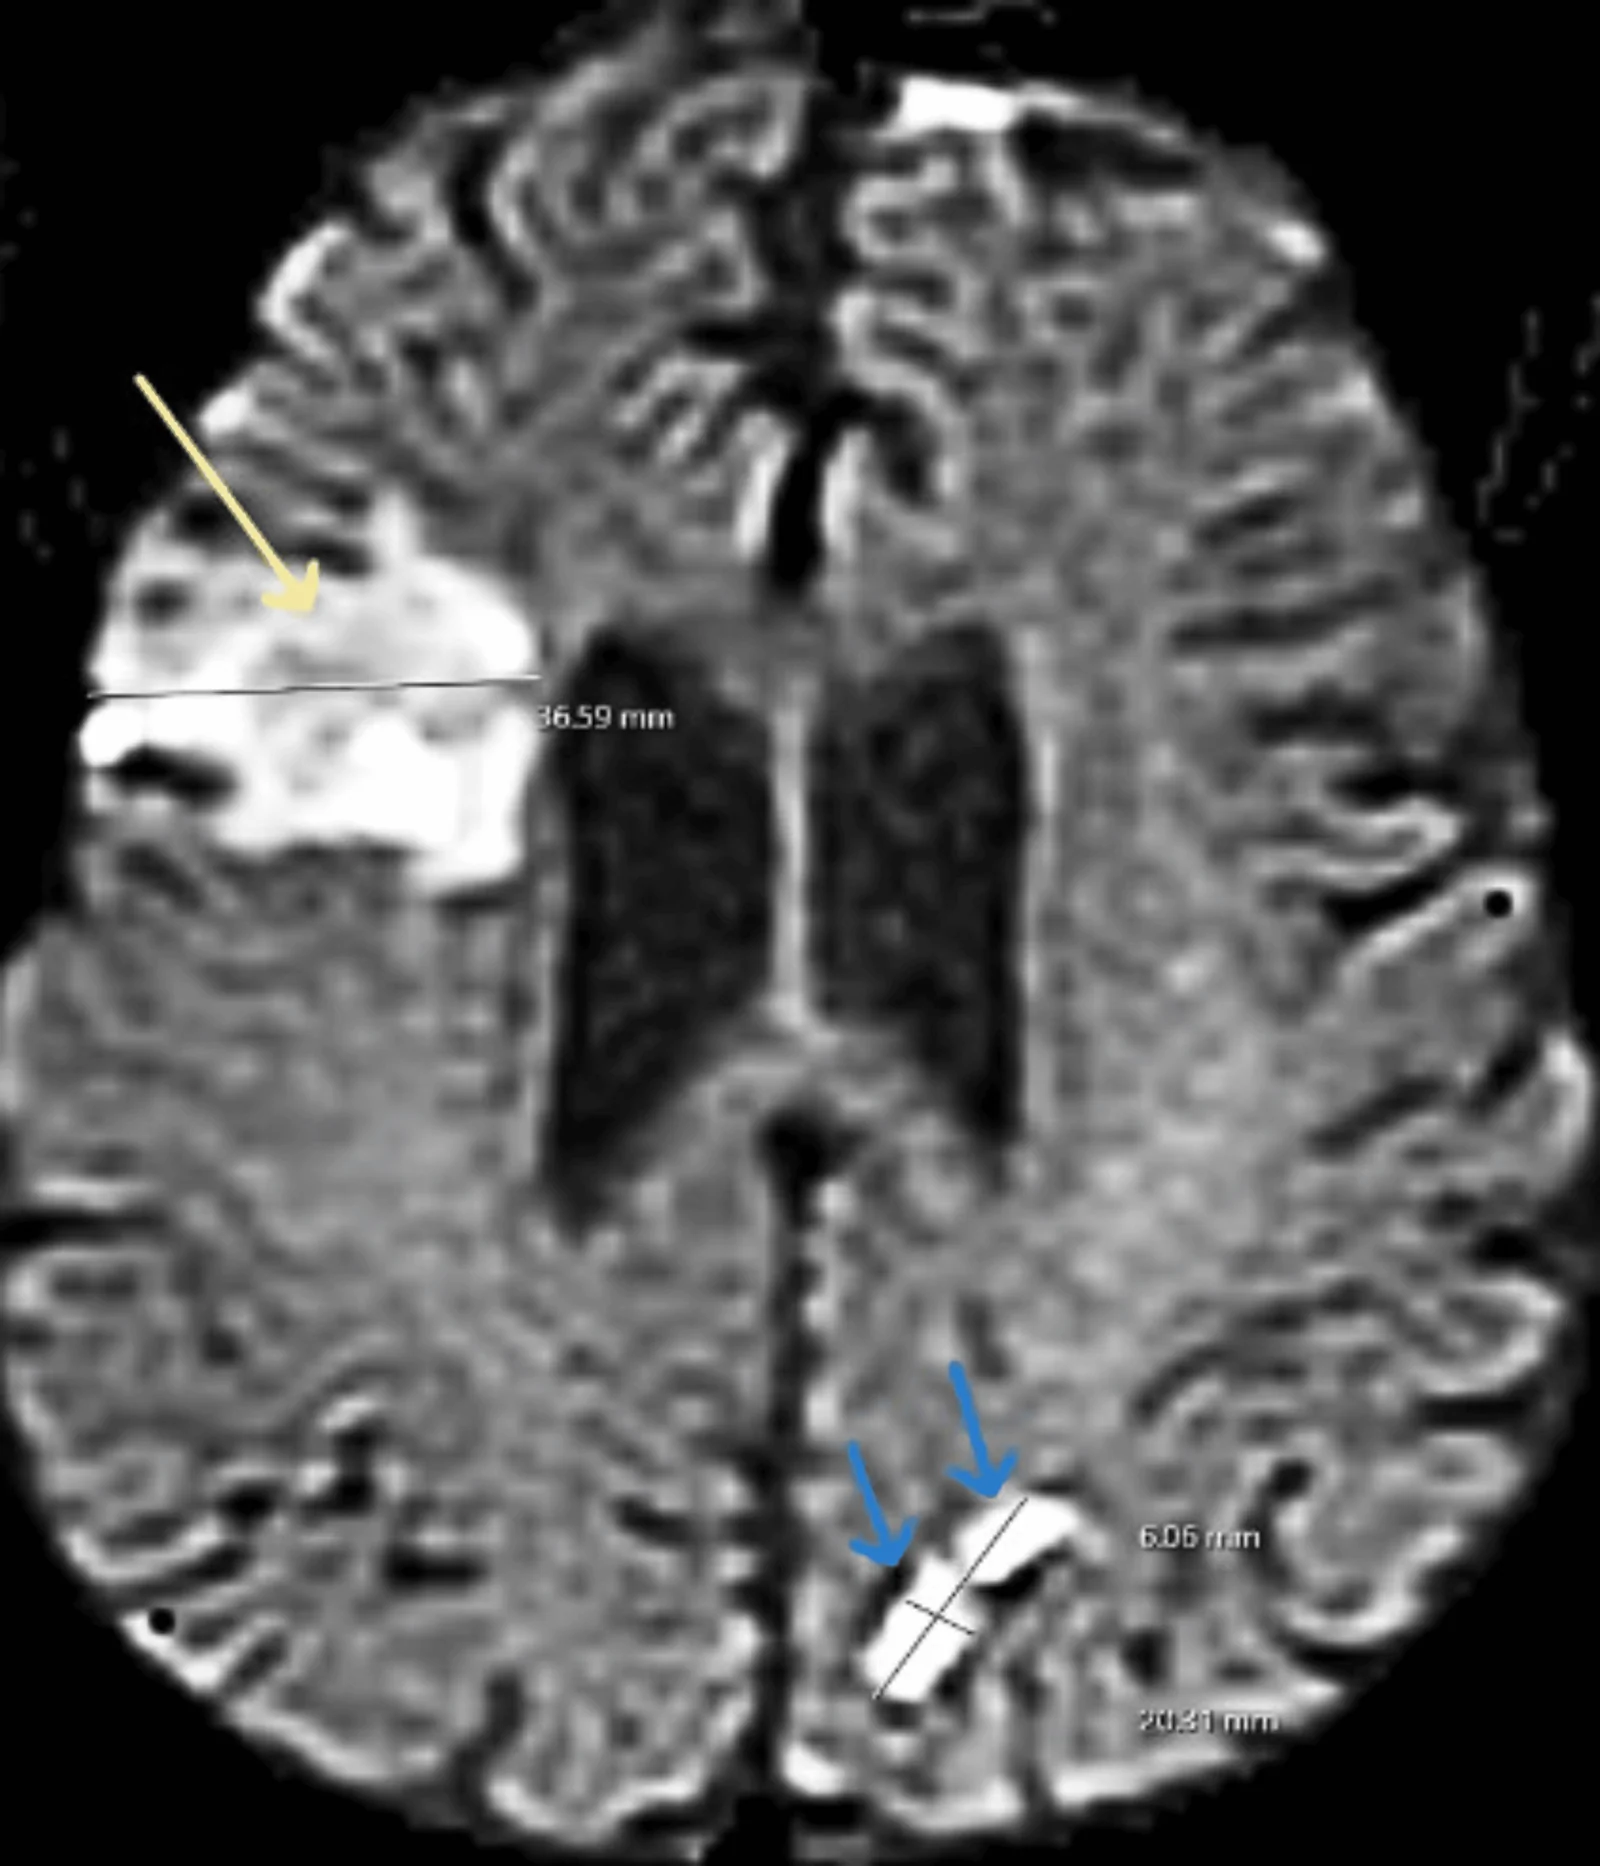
MRI of the brain on Day 4. DWI demonstrating restricted diffusion in the right frontal lobe consistent with a 36.59 mm subacute infarction (yellow arrow) and additional subacute infarctions in the left parietal lobe (blue arrows), confirming a bilateral embolic distribution MRI: magnetic resonance imaging; DWI: diffusion-weighted imaging
